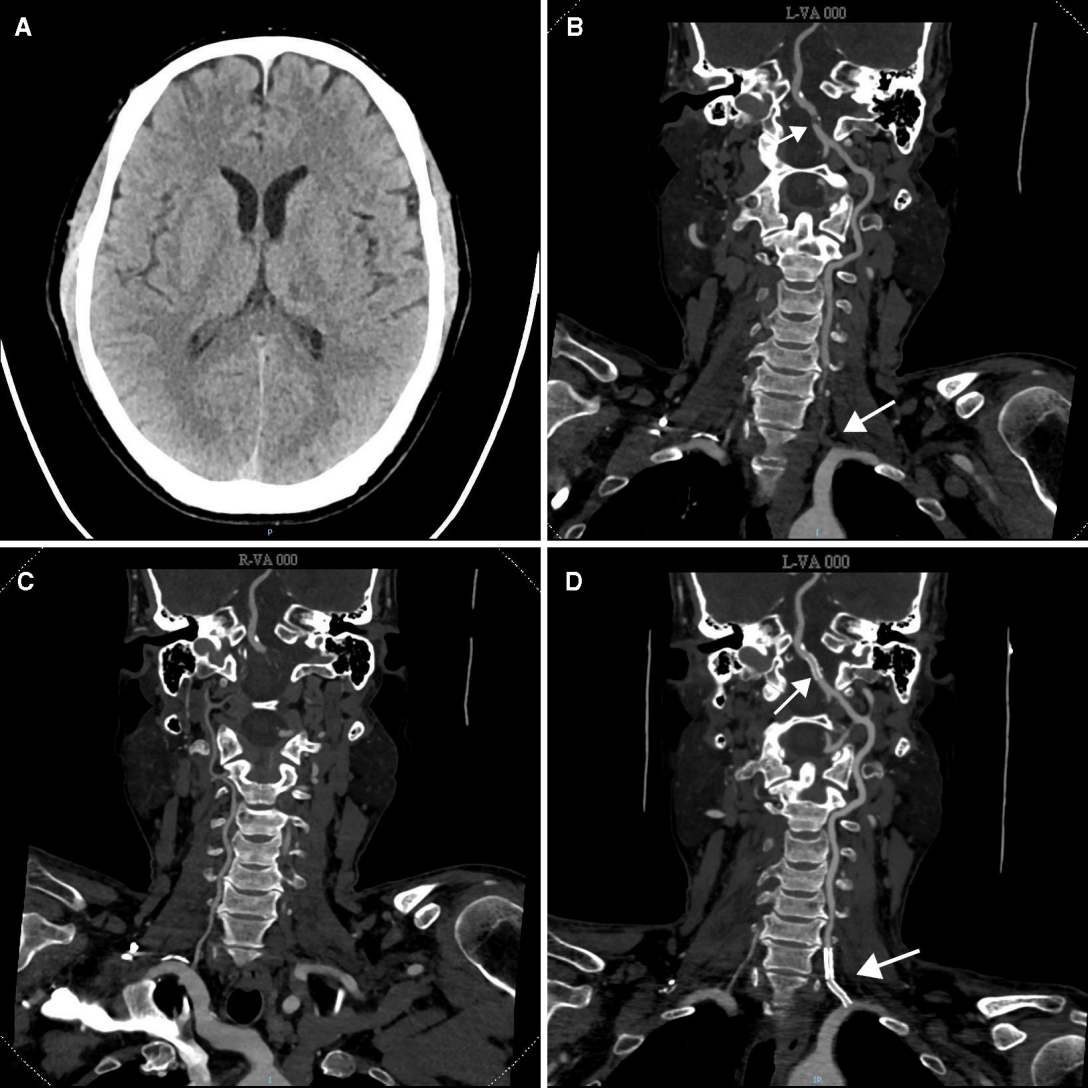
Pre- and post-treatment CT evaluation of the patient. (A) Pre-treatment non-contrast CT scan demonstrated a hypodensity lesion in the left thalamus. (B) Cervical and cerebral CT angiography (CTA) showed proximal occlusion with a non-tapered stump (white arrow) and distal severe stenosis of the left vertebral artery. (C) CTA showed that the right vertebral artery did not join in the basilar artery. (D) Post-treatment CTA indicated that the left vertebral artery was recanalized with stent placed inside (white arrow).
